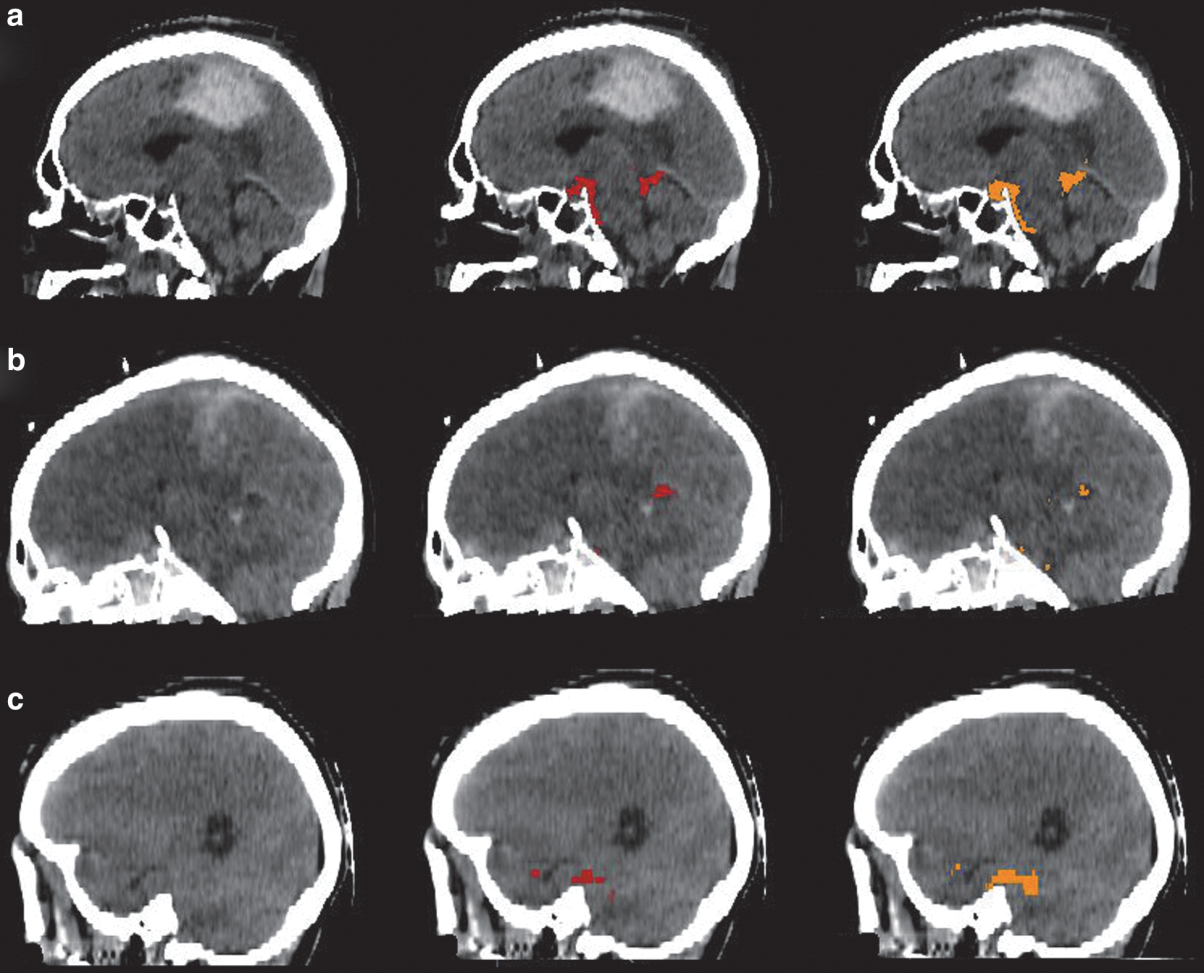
Qualitative results for cisterns segmentation on 3 representative subjects from data set 2. The first column shows original computed tomography images with superimposed cisterns segmentations from the expert in the second column and icobrain in the third column. (A) Cisterns segmentation on a representative subject with Dice of 0.73, followed by (B) Dice of 0.70 and (C) Dice of 0.66. Color image is available online.
